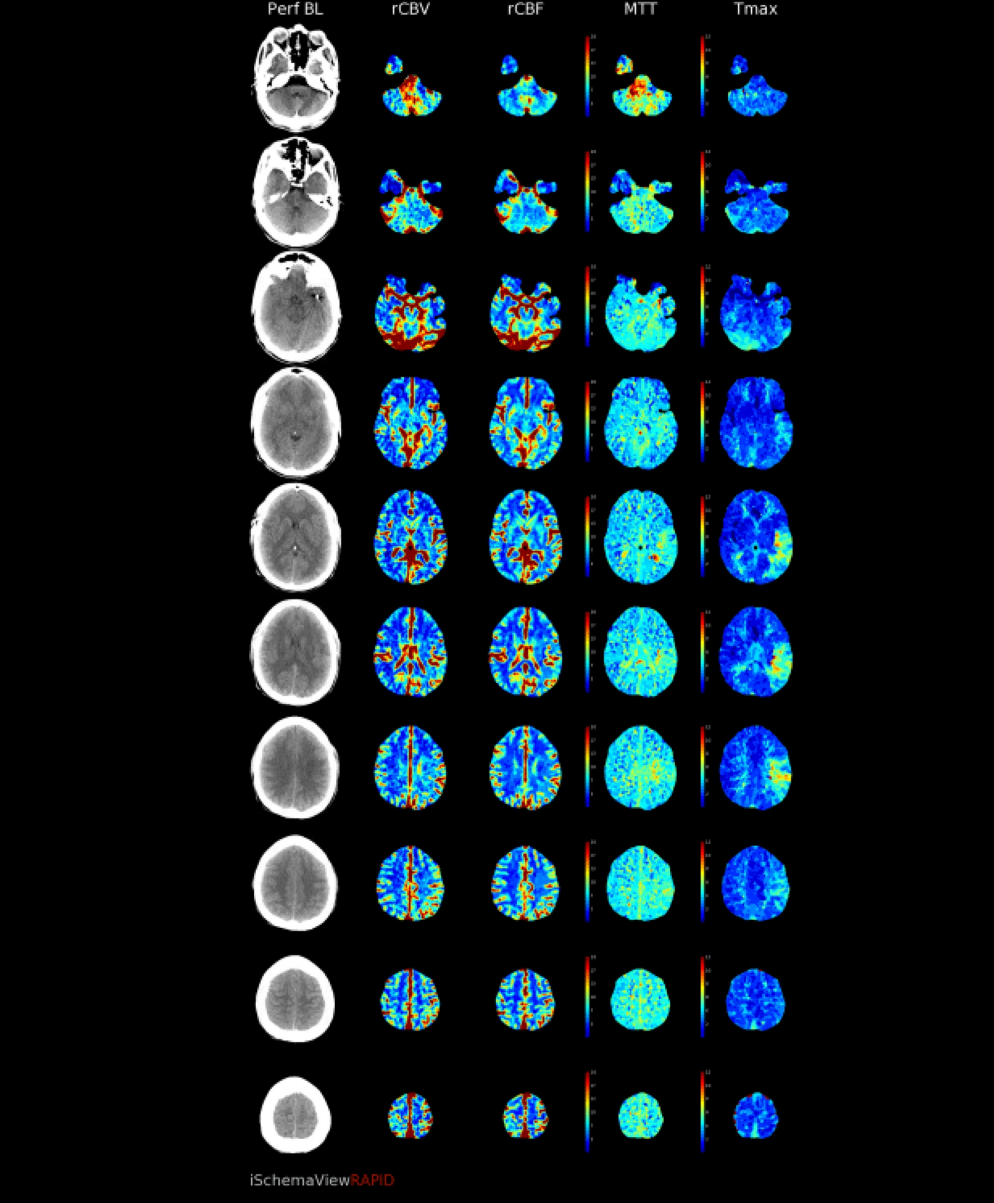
CT perfusion demonstrating preserved rCBV, rCBF, and MTT with elevated Tmax in the left MCA territory of the left frontal lobe
